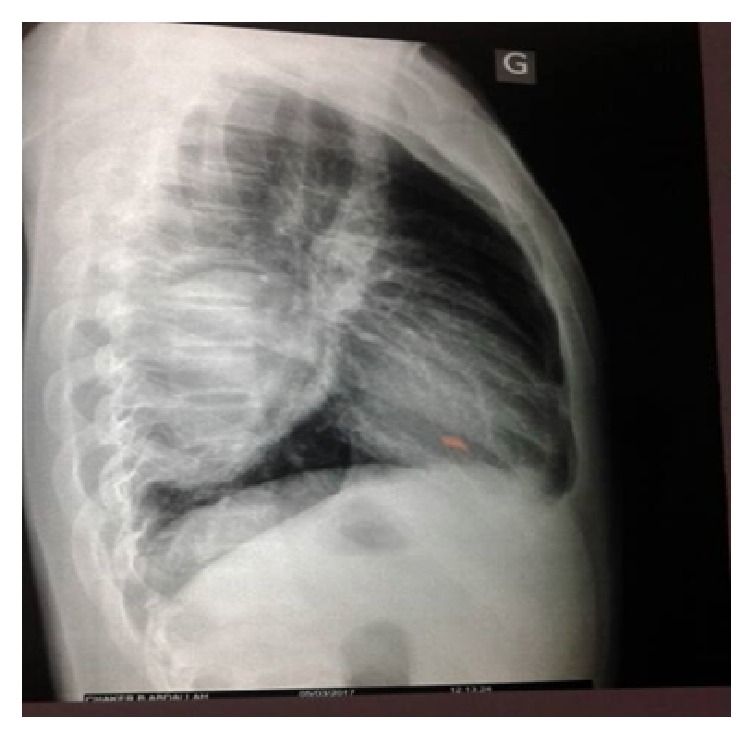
Lateral view chest X-ray showing a well-defined rounded opacity was in the retrocardiac space.
